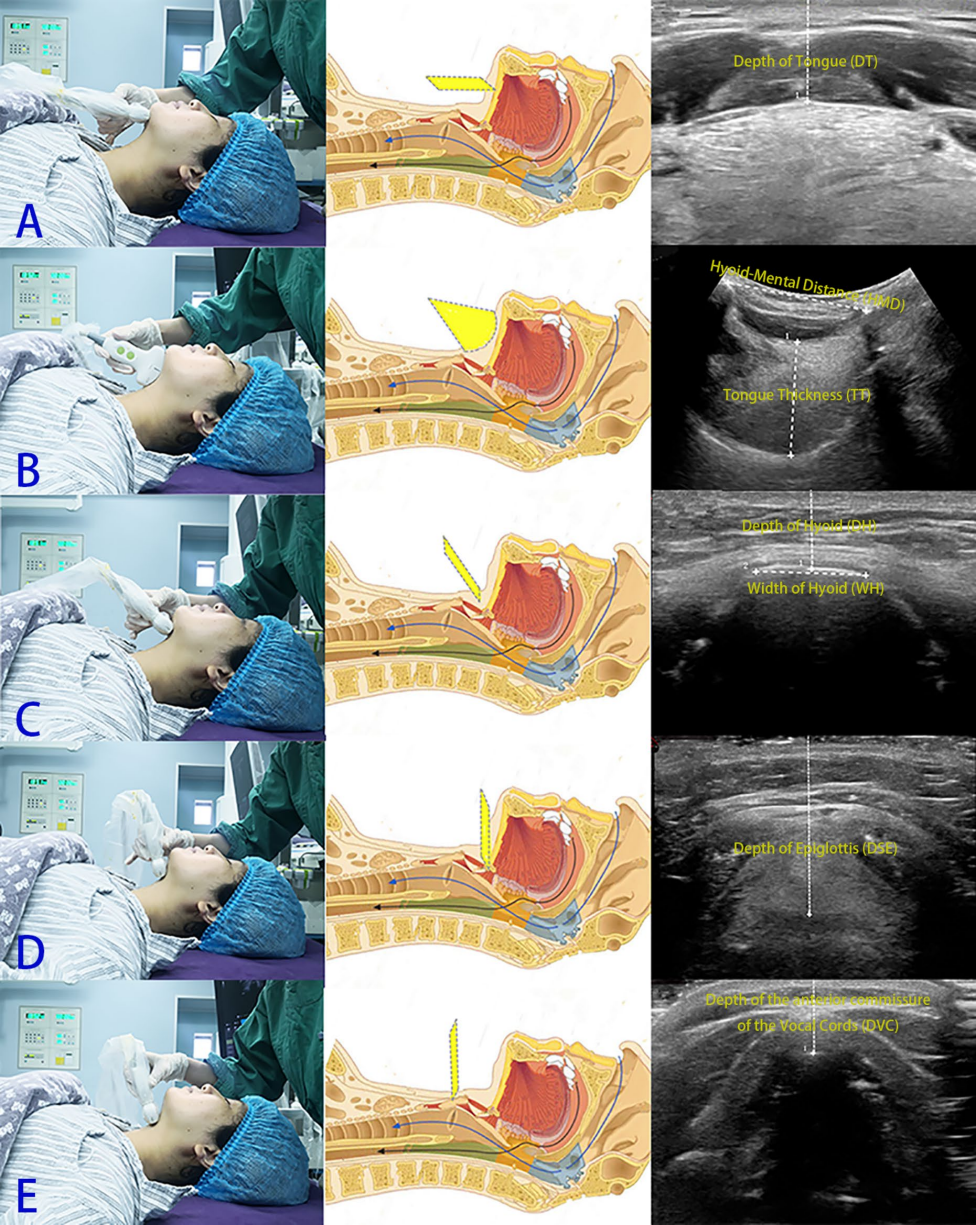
Patient positioning and transducer placement for measuring (left), anatomical (middle) and ultrasonography views (right) at the level of the tongue surface (A), tongue body (B), hyoid (C), epiglottis (D) and vocal cords (E), before anaesthetic induction. Consent to publish the figure was obtained from the patient.(2)Thickness of the thickest part of Tongue body (Tongue Thickness, TT) and Hyoid-Mental Distance (HMD), Fig. 1B^14,15^.(3)Distance from Hyoid to skin (Depth of Hyoid, DH) and the Width of Hyoid (WH), Fig. 1C^14^.(4)Distance from Skin to Epiglottis (Depth of Epiglottis, DSE), Fig. 1D^16^.(5) Depth of the anterior commissure of the Vocal Cords (DVC), Fig. 1E^14^. The images had been stored on the hard drive of the ultrasound device (Fig. 1).
